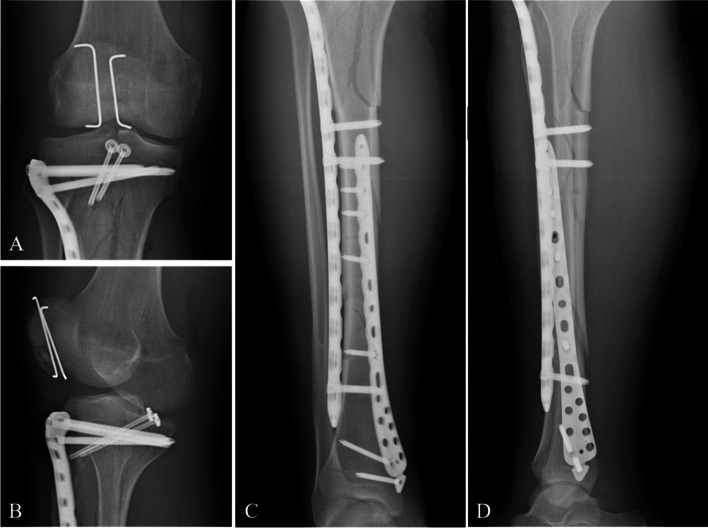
Postoperative radiographs: AP (A) and oblique (B) views of the right knee showed that the PCLTAF was treated through two partially threaded cancellous lag screws with washers, the patellar fractures were treated by ORIF using Kirschner wires, and the proximal tibial plateau fractures were managed by applying locked plates and screws. AP (C) and lateral (D) views of the tibia revealed that the tibial shaft fractures were managed using locked plates and screws
